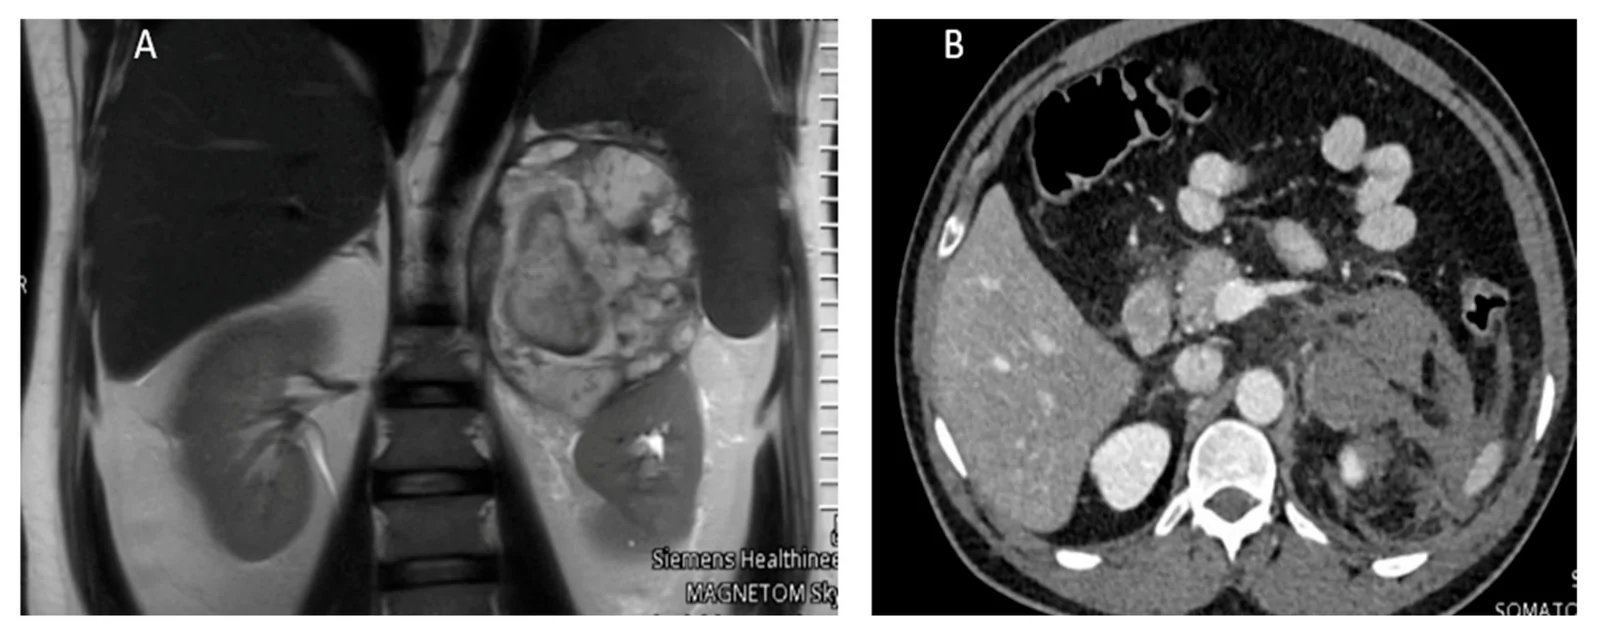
CT scans of abdomen and pelvis with oral and IV contrast plain and at porto-venous phase: (A) and (B): There is large dense lesion in the left adrenal gland measuring 5 × 4 cm in size with extension and a continuation of a retroperitoneal soft tissue dense lesion, which extends to lower abdomen and measures approximately 14 × 5.5 cm in size, with significant surrounding fat stranding after contrast injection. There is a notable rim enhancement of the remaining peripheral left adrenal gland (Case 2).
